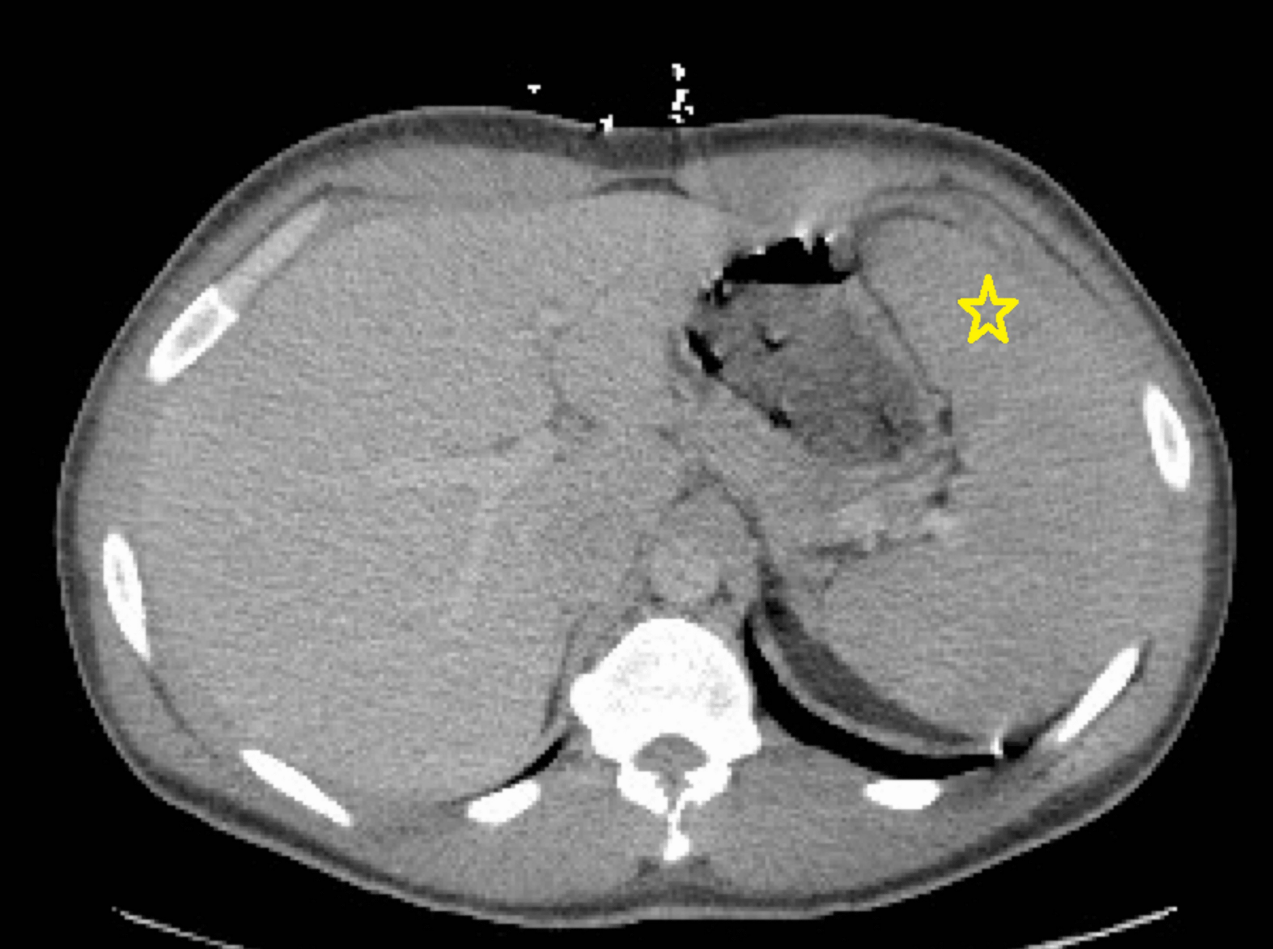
Computed tomography demonstrating splenomegaly The star indicates the spleen.
